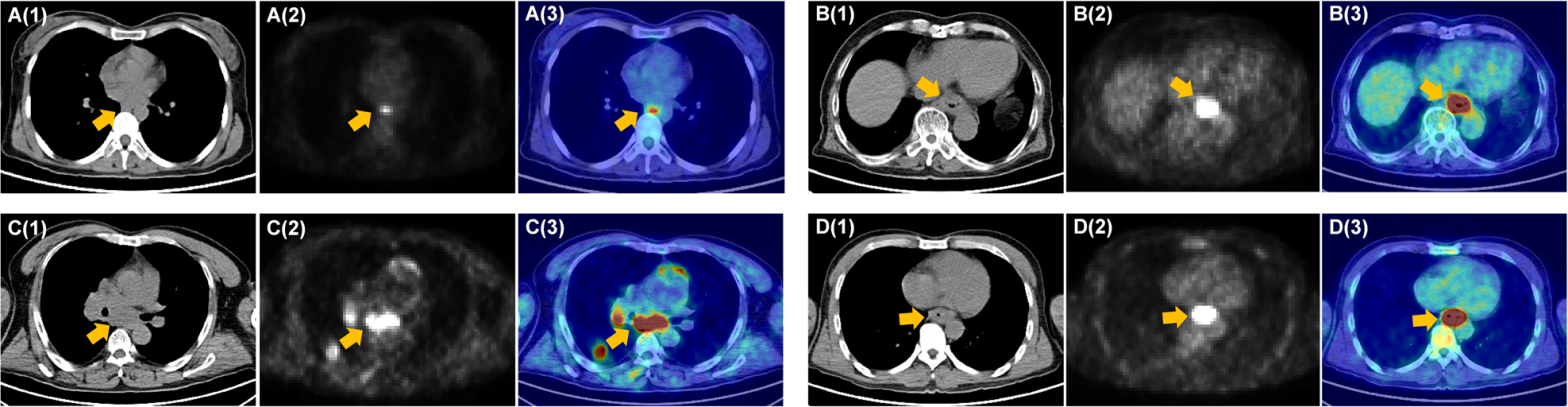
CT, PET, and PET/CT fusion images of two patients who underwent SA: A A 38-year-old woman with low-differentiated squamous cell carcinoma in the middle of the chest (ESCCPro-SA score: 0.11, OS: 26.6 months). B A 74-year-old man with low-differentiated squamous cell carcinoma in the lower thoracic segment (ESCCPro-SA score: 0.95, OS: 3.0 months). Two patients underwent surgical resection plus postoperative adjuvant chemotherapy: C A 68-year-old man with moderate-differentiated squamous cell carcinoma in the middle of the chest (ESCCPro-SPOCT score: 0.09, OS: 100.0 months). D A 51-year-old man with moderate-differentiated squamous cell carcinoma in the lower thoracic segment (ESCCPro-SPOCT score: 0.75, OS: 15.1 months)
